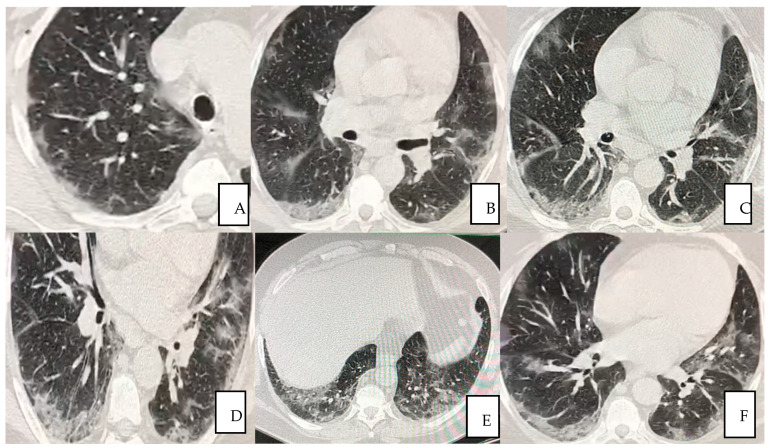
(A–F) A 56-year-old man presented with fever for 3 days, accompanied by anuria, asthenia, general malaise and positive to COVID-19 (A–E) Pulmonary HRCT obtained on 18 November 2020 showed multiple peripheral patchy ground glass opacities bilaterally being the lower lobes the most involved areas in (F).
